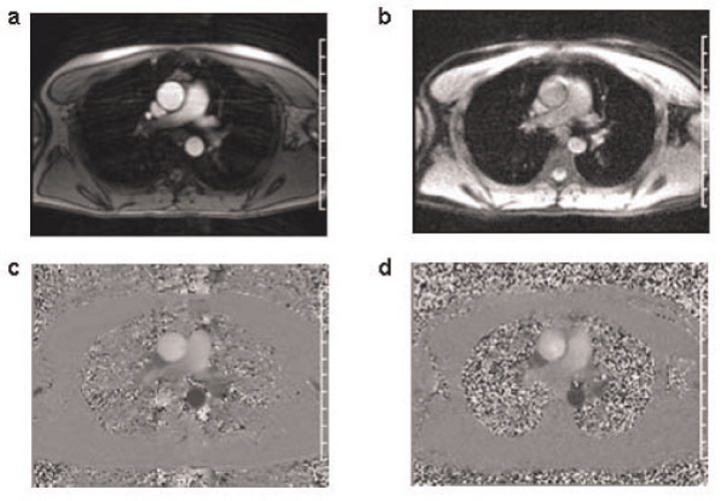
Magnitude images acquired using (a) conventional segmented, (b) real-time GRE-EPI SVE PC-MRI, and phase velocity maps from (c) conventional segmented PC-MRI, and (d) real-time GRE-EPI SVE PC-MRI sequence in a slice cutting the ascending and descending aorta close to the aortic arch.
